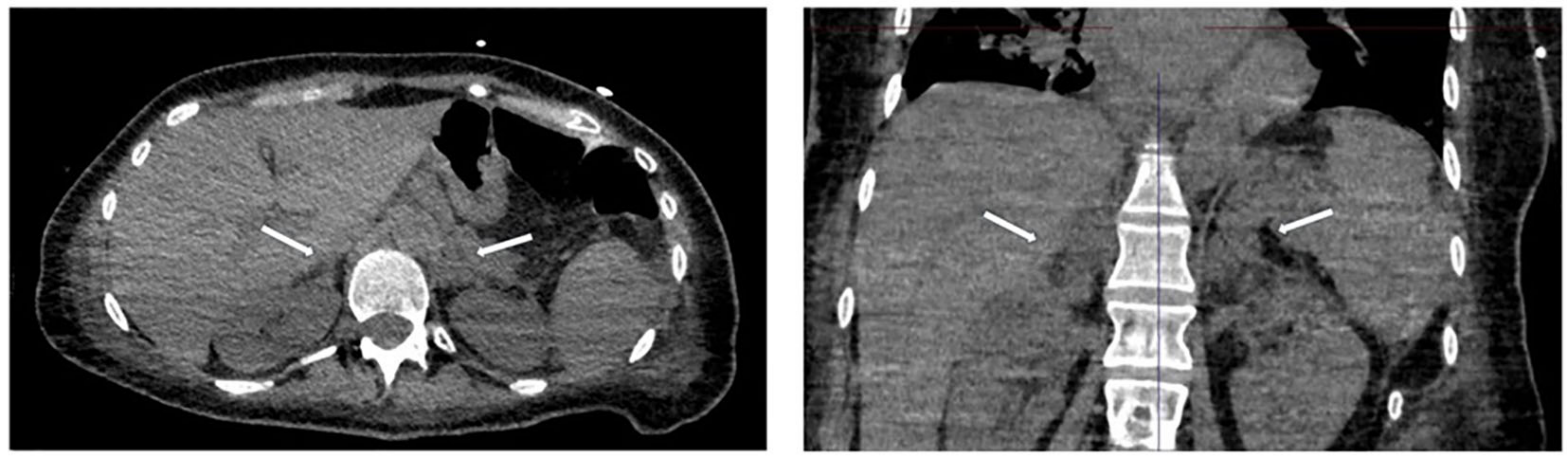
Transverse (left) and coronal (right) CT images of the abdomen, showing bilateral adrenal hemorrhage.
